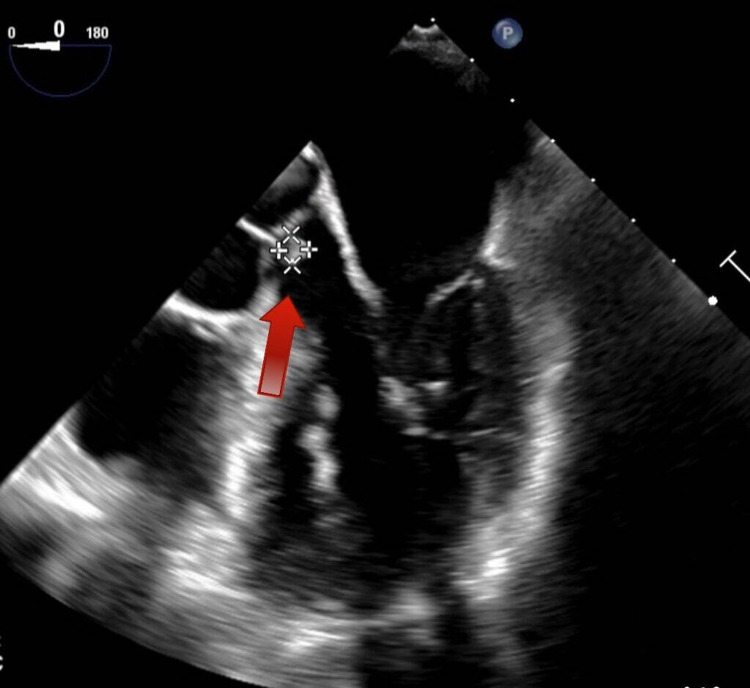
Transesophageal echocardiogram (TEE) showing an echogenic mass (red arrow) that represents the septic vegetation attached to the aortic valve cusp
